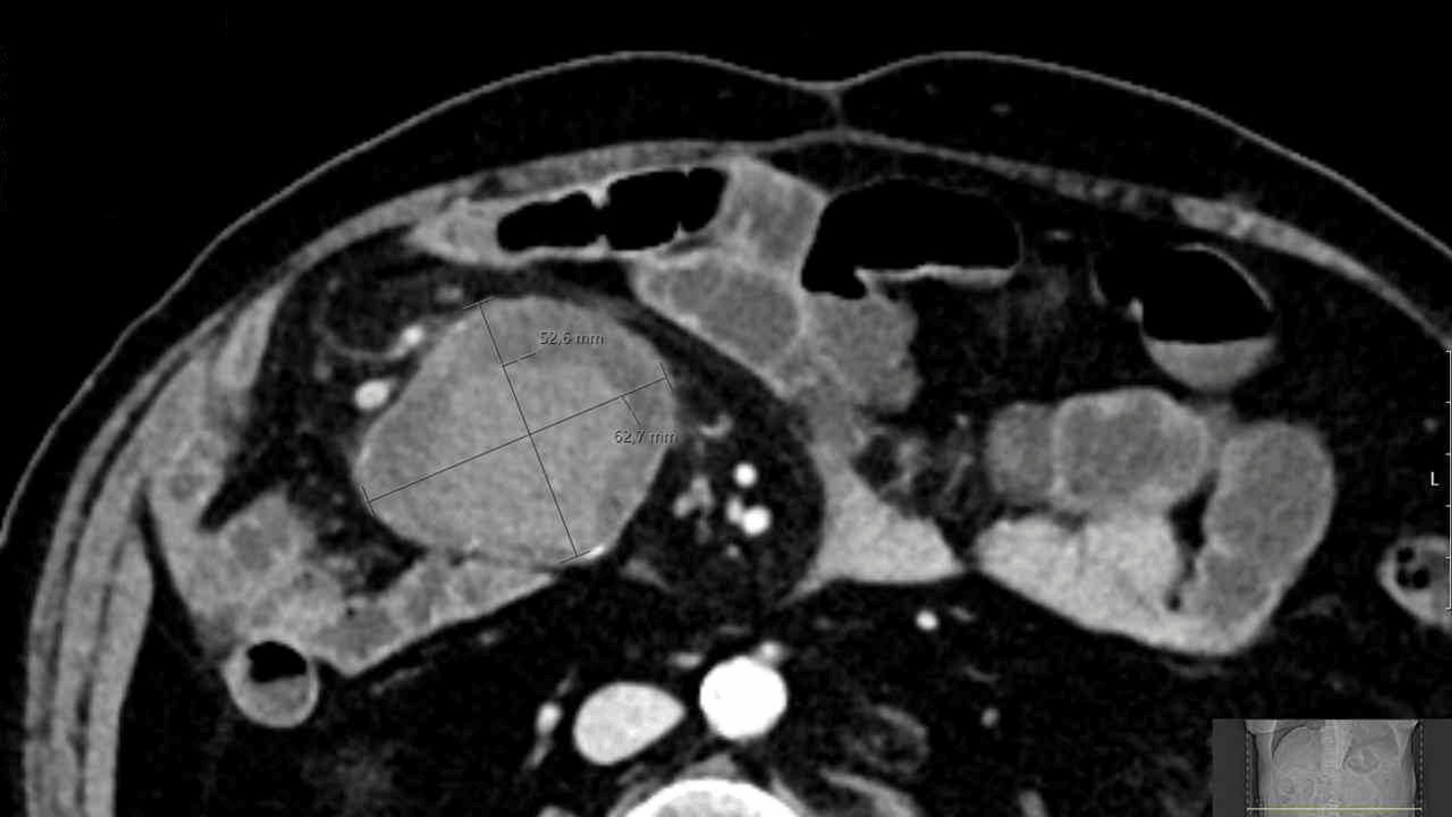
CT scan showing a solid, inhomogeneous mass in the right side of the mid-abdomen measuring 6.3 × 5.3 × 4.8 cm.
